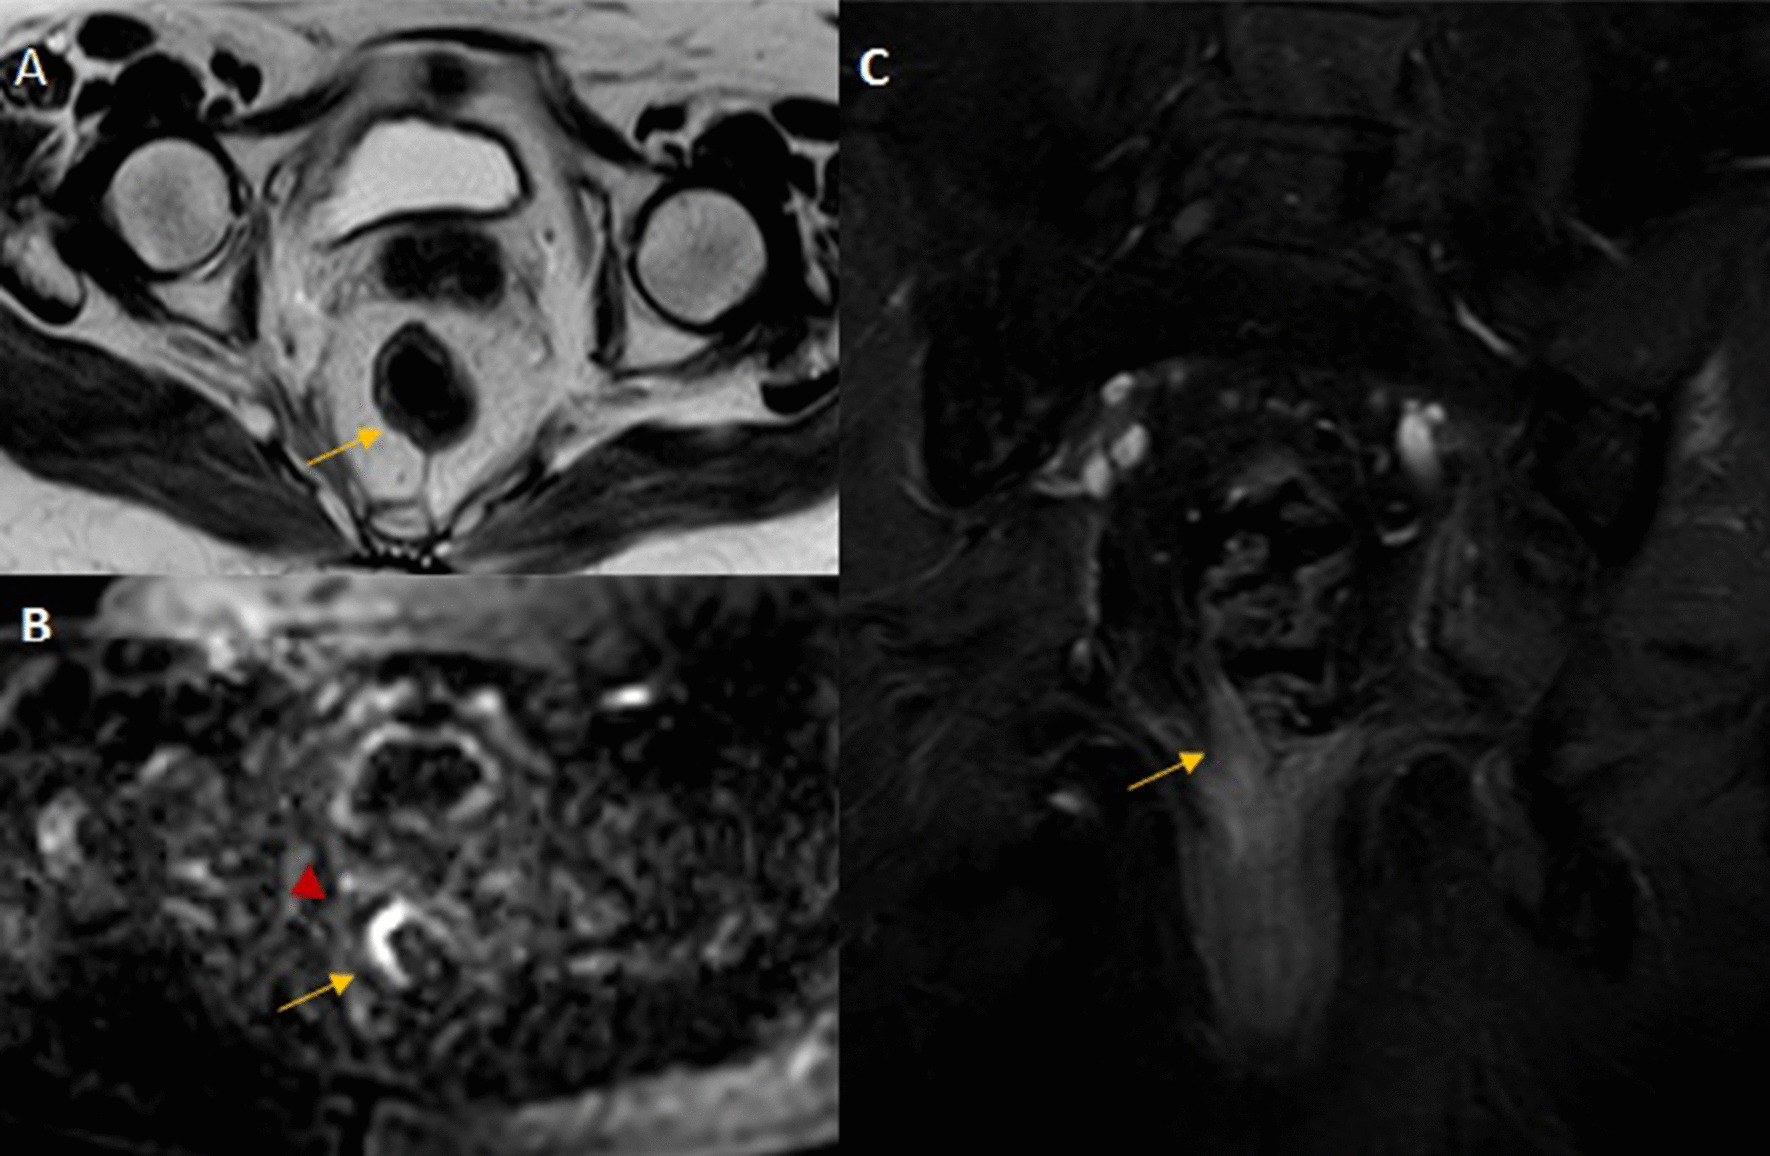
Pre and post contrast pelvic MRI: 52-year-old female post neoadjuvant rectal adenocarcinoma: Axial T2 weighted image (A), Axial diffusion weighted image (B) and coronal post contrast T1 weighted image with fat suppression (C): Focal irregular mural thickening of the lower third rectum from 6 to 12 o’clock (arrows) with intact external muscle layer, no extramural spread or MRF involvement, no downward extension into the anal sphincters. Tiny mesorectal rounded LN (arrowhead) better detected on DW image, no extra-mesorectal LNs. MRI based staging T2, N1
